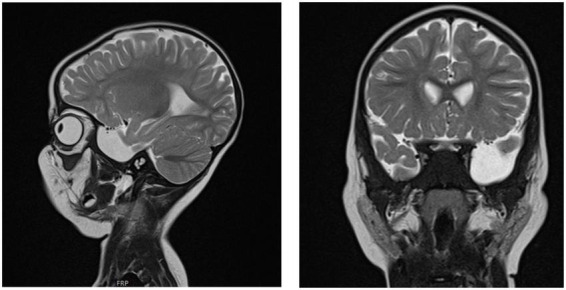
Brain MRI of patient six at the age of 2 years. T2 sagittal and coronal MRIs show an arachnoidal cyst in the left temporal region and atrophy of the left temporal lobe.
